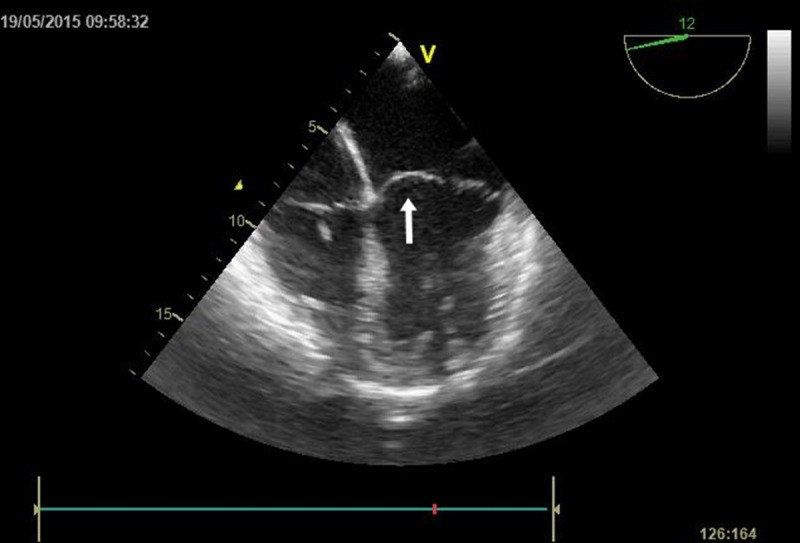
Transesophageal echocardiographic preoperative assessment of MVP showing prolapsed mitral leaflets (arrow) over the annular plane of at least 2 mm.
